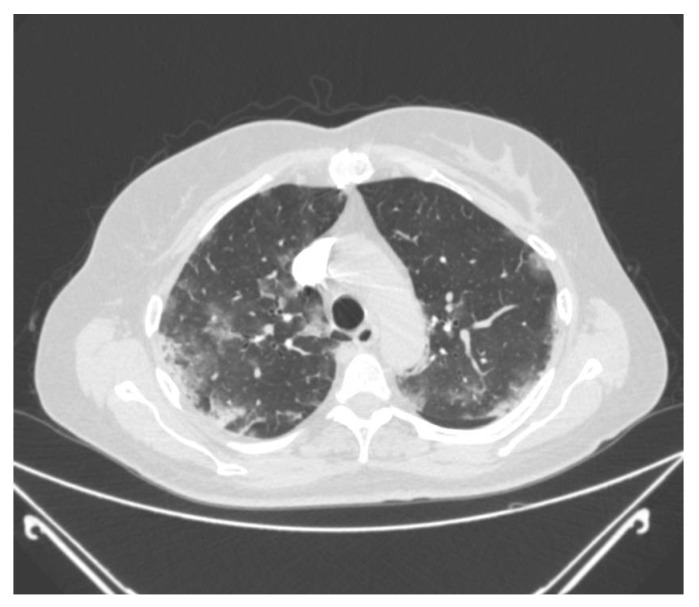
Ground-glass opacities on chest CT in a patient with COVID-19-related pneumonia.
